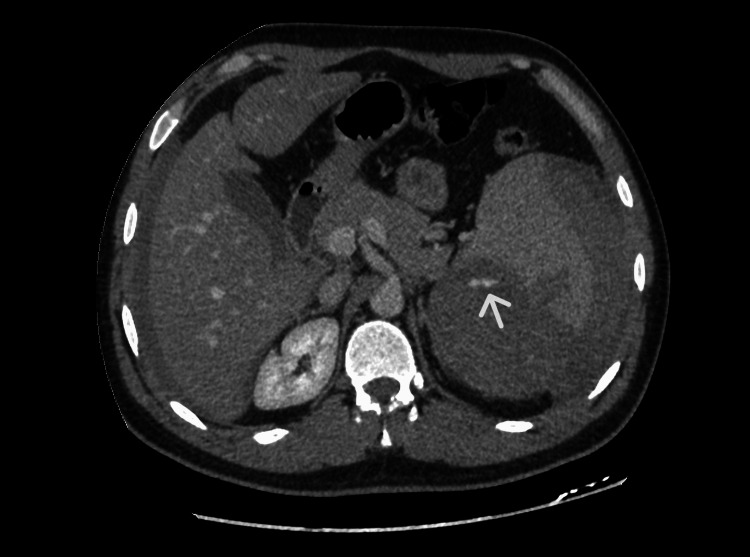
Enhanced CT shows areas of contrast enhancement seen more posterior and inferior to the spleen and adjacent to the splenic hypodensity
